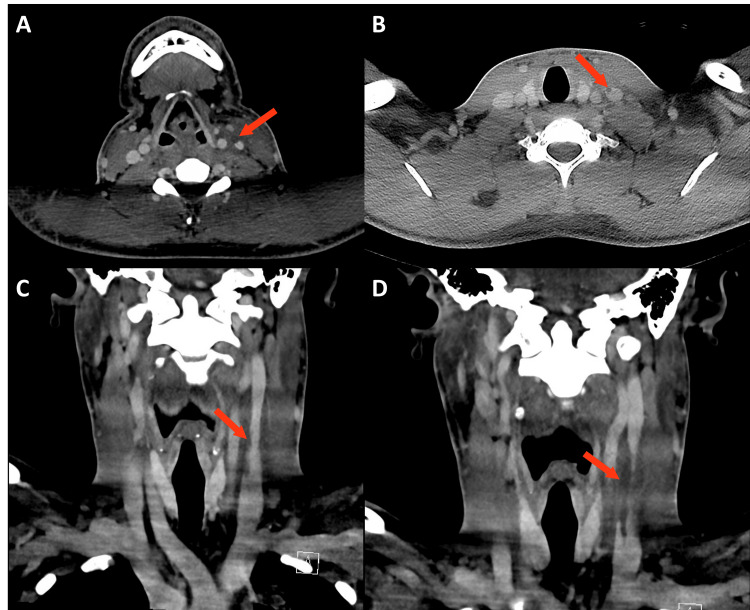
CT Soft Tissue Neck With Thrombus in the Left Internal Jugular Vein CT soft tissue neck with contrast showing a filling defect within the left internal jugular vein consistent with a moderate thrombus (arrows). Diffuse fat stranding is also noted throughout, suggesting a degree of anasarca.  No focally compressive phlegmons or abscesses are noted.
